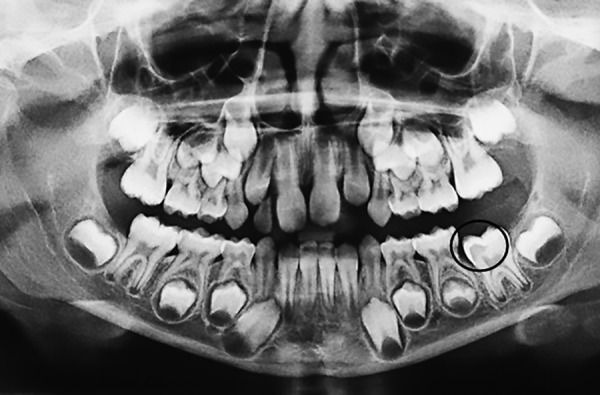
Panoramic radiograph showing a radiolucent area on the coronal portion of the left mandibular first permanent molar of the patient
